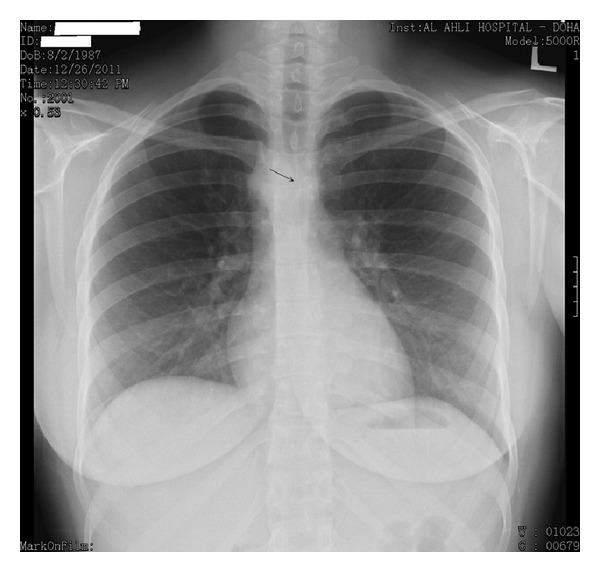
A posteroanterior view of chest X-ray showing an abnormal distal tracheal air shadow (arrow) and the absent left aortic knuckle.
